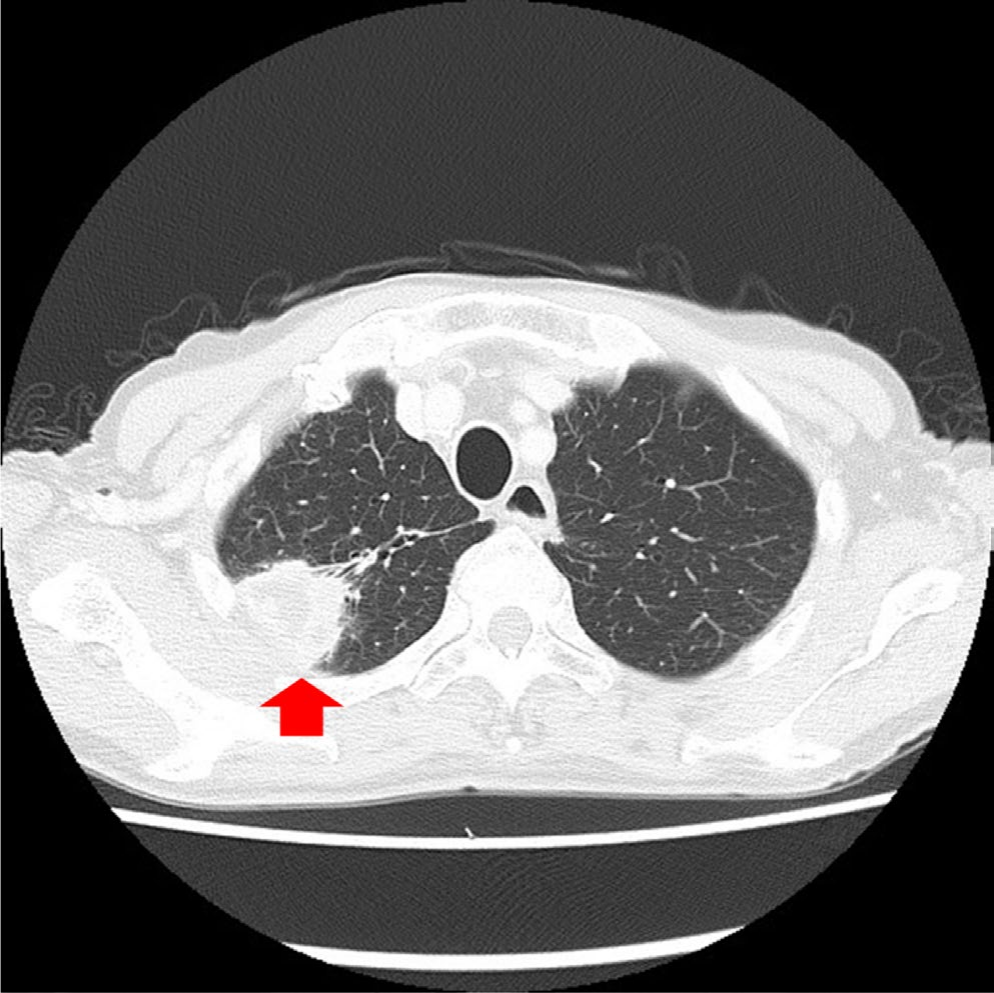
Chest computed tomography (CT) scan of an 81‐y‐old male showing a mass on the right pulmonary apex, which was diagnosed as a lung abscess caused by *α‐Hemolytic streptococci*, *Neisseria* spp., *Haemophilus* spp., and *Serratia fonticola*
